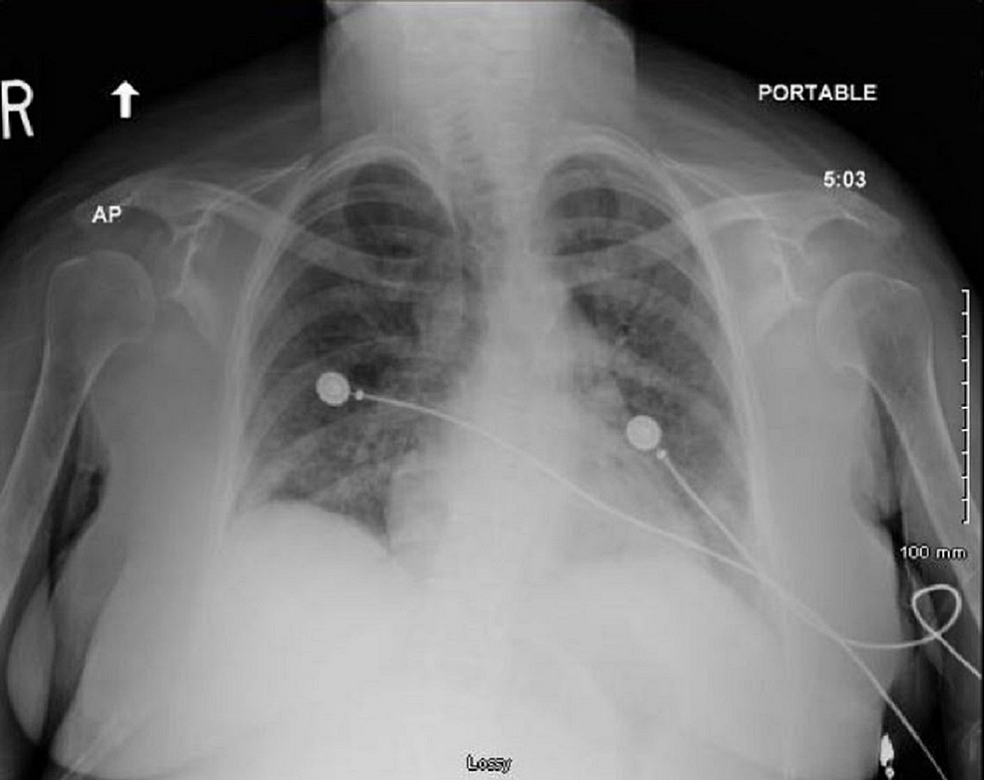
Chest X-ray of the patient showing diffuse and bilateral interstitial edema.
